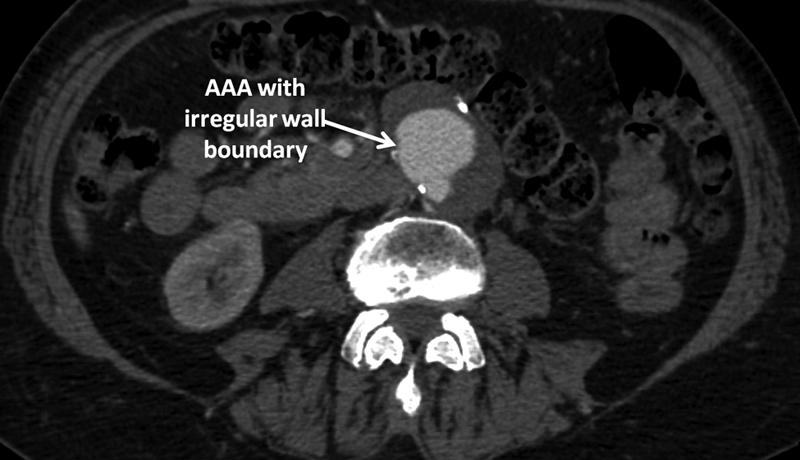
Axial computed tomography image. AAA, abdominal aortic aneurysm.
